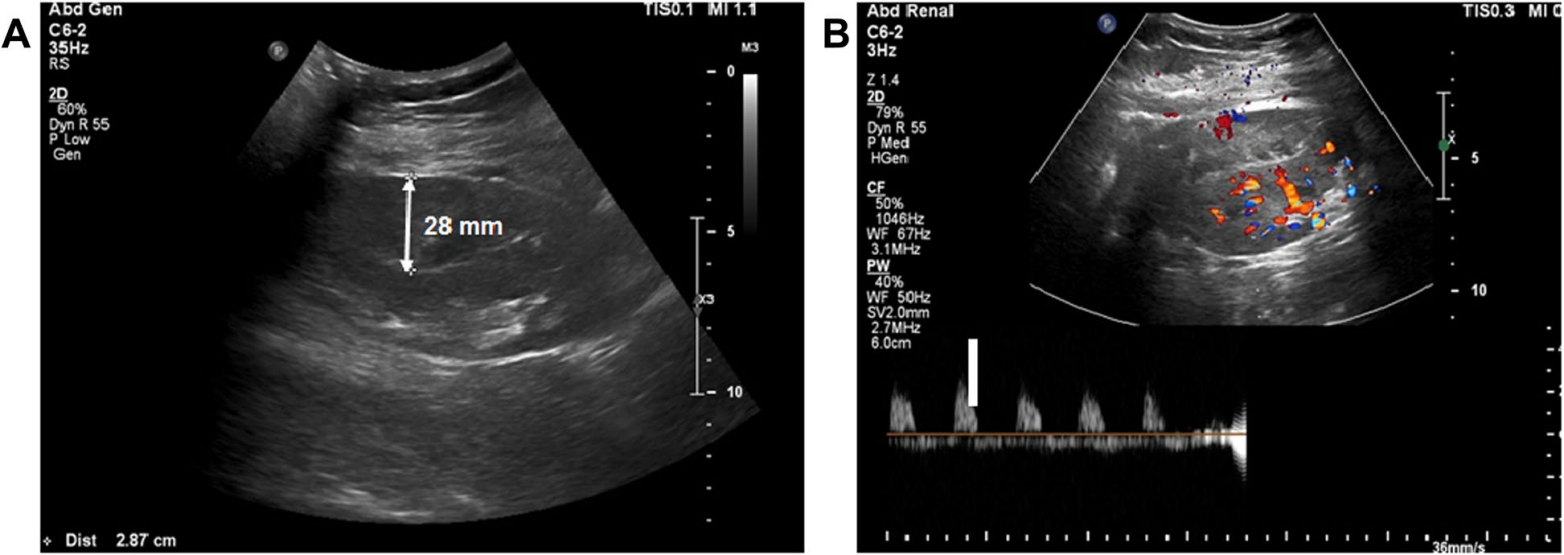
Doppler USG showing 28 mm in depth hematoma (A) and diastolic flow reversal (resistive index = 0.9–1) (B)
